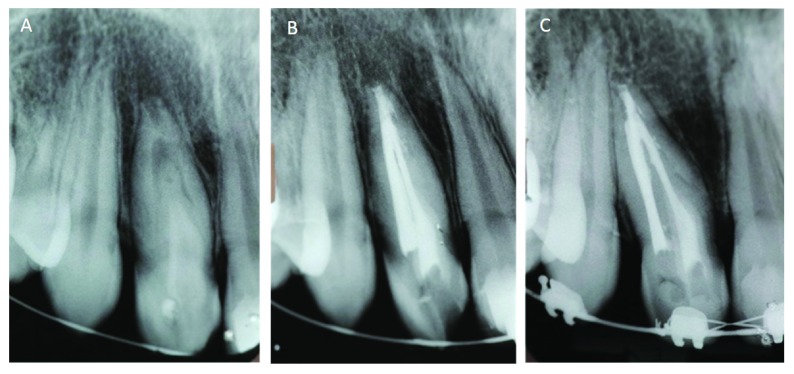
Radiographic examinations of case 2. (
